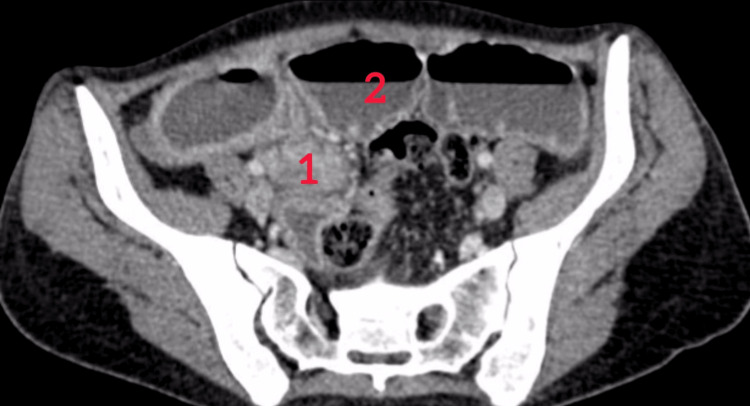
Axial CT image showing small bowel hydro-aeric distension below a stricture of the terminal ileum. (1) Ileal stricture. (2) Distension of the ileum.
